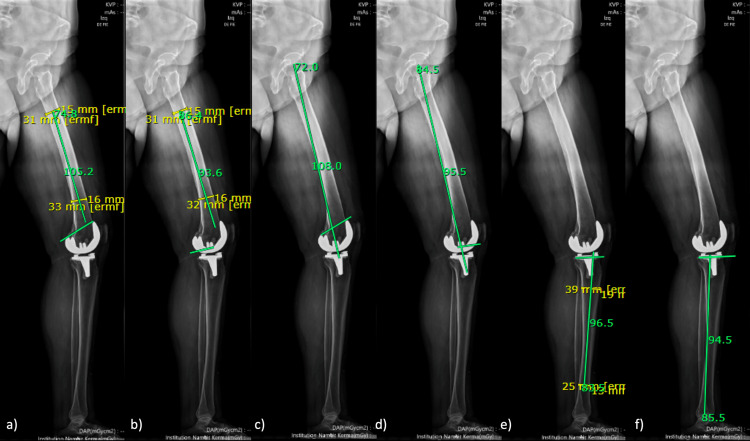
Full-length standing lateral radiograph (a)Sagittal anatomic femoral component alignment 1; (b) Sagittal anatomic femoral component alignment 2; (c) Sagittal mechanical femoral component alignment 1; (d) Sagittal mechanical femoral component alignment 2; (e) Sagittal anatomic tibial component alignment; (f) Sagittal mechanical tibial component alignment
